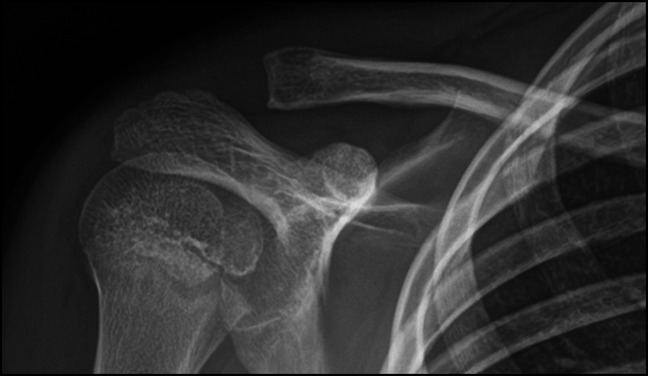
Anteroposterior radiograph of the right shoulder demonstrating a lytic lesion with cortical thinning affecting the distal clavicle.
